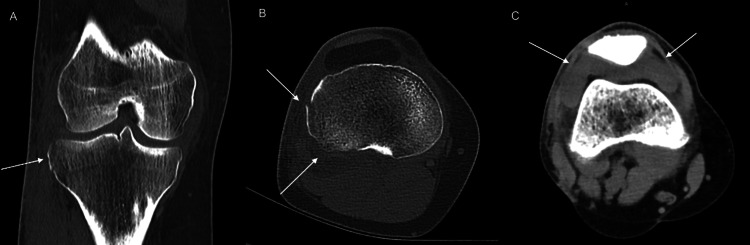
(A) Coronal CT image shows the Segond fracture with a craniocaudal size of 16 mm (arrow). (B) Axial CT image in bone window shows the Segond fracture with a maximum anterior-posterior size of approximately 20 mm as well as cortical incongruence of the dorsolateral tibia plateau as an impression fracture (arrows). The Segond fracture has a minimal lateral displacement. Additional fractures of the knee were ruled out. (C) The axial CT image in soft tissue window shows hemarthrosis in the knee joint (arrows). CT: computed tomography
